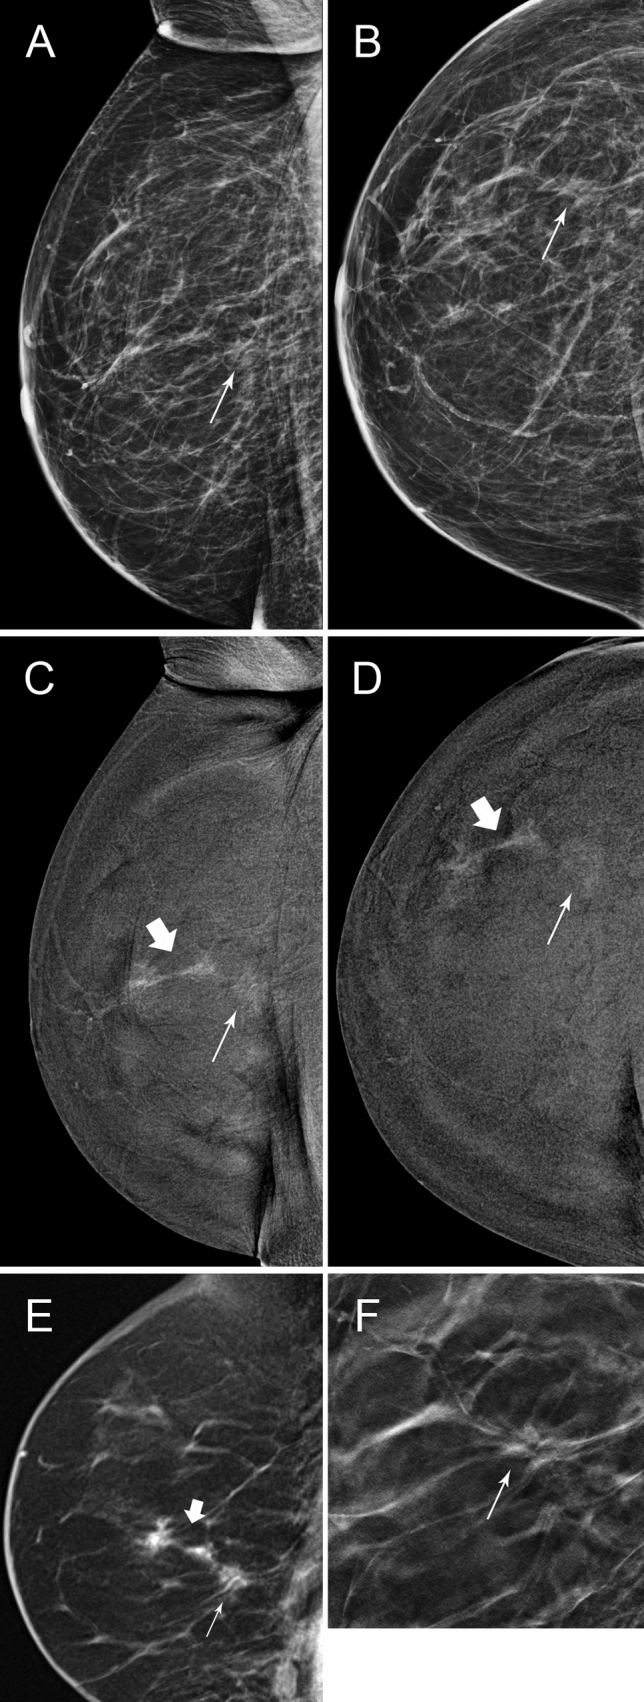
A and B, 72 year-old patient, 2D 2019 surveillance images, MD BIRADS B. She had right wide local excision 2009 for a small Gd 3 invasive node-positive cancer. Thin arrow shows a small spiculated density thought to be part of the scar, stable on tomography. F is the CC tomographic image of this density(thin arrow). C and D are C + images from the same surveillance study showing faint enhancement (thin arrow) in the spiculated density, but a further 35 mm of spiculated enhancement anteriorly (thick arrow), not seen on 2D, 3D or targeted ultrasound. E is a sagittal contrast enhanced MR image (thin MIP) at the time of MR guided biopsy showing excellent correlation of CEM & MR images. Right mastectomy showed invasive carcinoma NST, Grade 3 with 2 tumours 22 &14 mm, triple negative, Ki67 20%. SNB negative. This was classified as a minimal signs case on 2D/3D after re-reading
